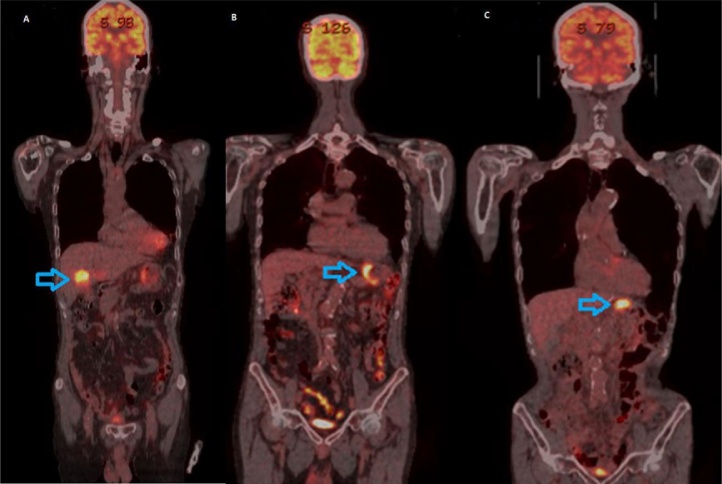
Positron emission tomography–computed tomography (PET-CT). A. Colon cancer (SUV; 12.6) without evidence of distant metastasis (cT3N0M0). B. Gastric cancer (SUV; 11.8) in upper body of stomach without evidence of distant metastasis (cT3N1M0). C. Local recurrence (SUV; 9.4) of previous stomach cancer without metastasis.
